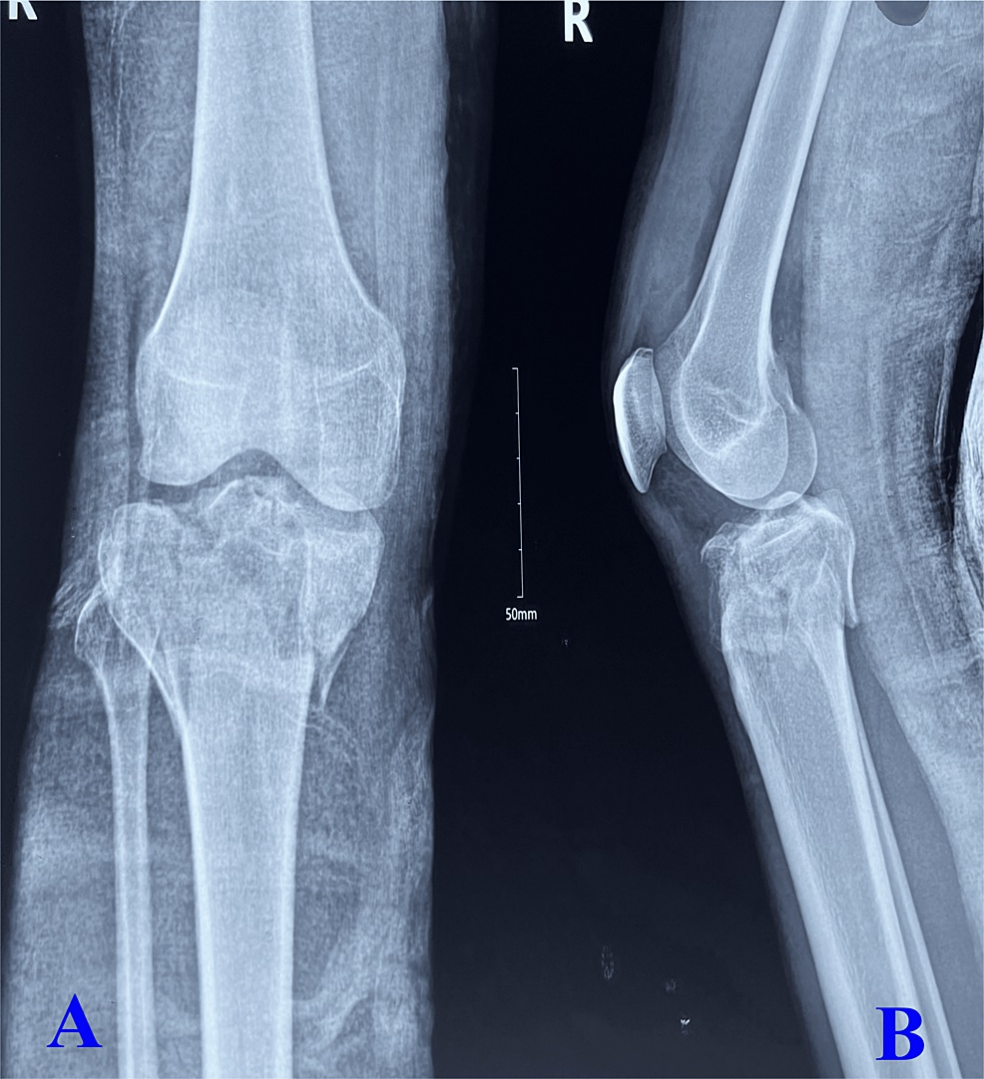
Preoperative X-rays of Schatzker type VI fracture showing the anteroposterior (A) and lateral (B) views A: The anteroposterior view shows a fracture of the medial and lateral column of the proximal tibia with metaphyseal and diaphyseal discontinuity. B: The lateral view shows a fracture of the posterior segment/part of the proximal tibia.
